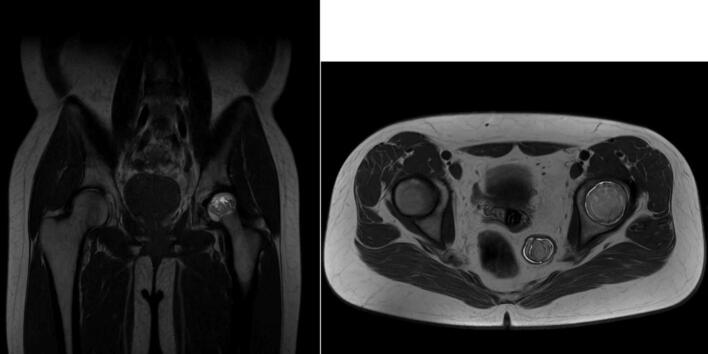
Pre-operative MRI coronal and axial view for patient III.
